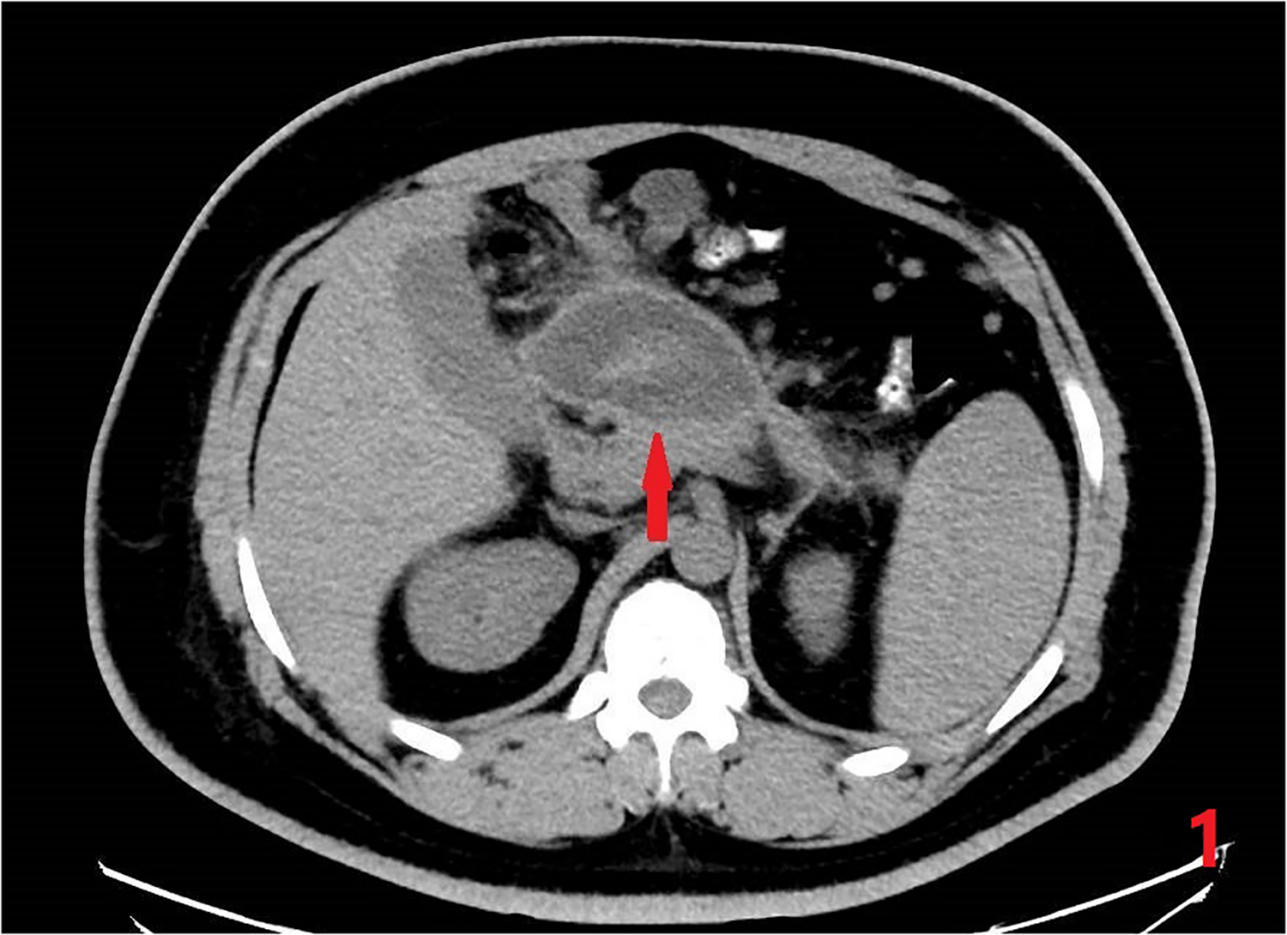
Preoperative CT. Pancreatic pseudocyst measuring 9.8*7.6*9.0 cm.
